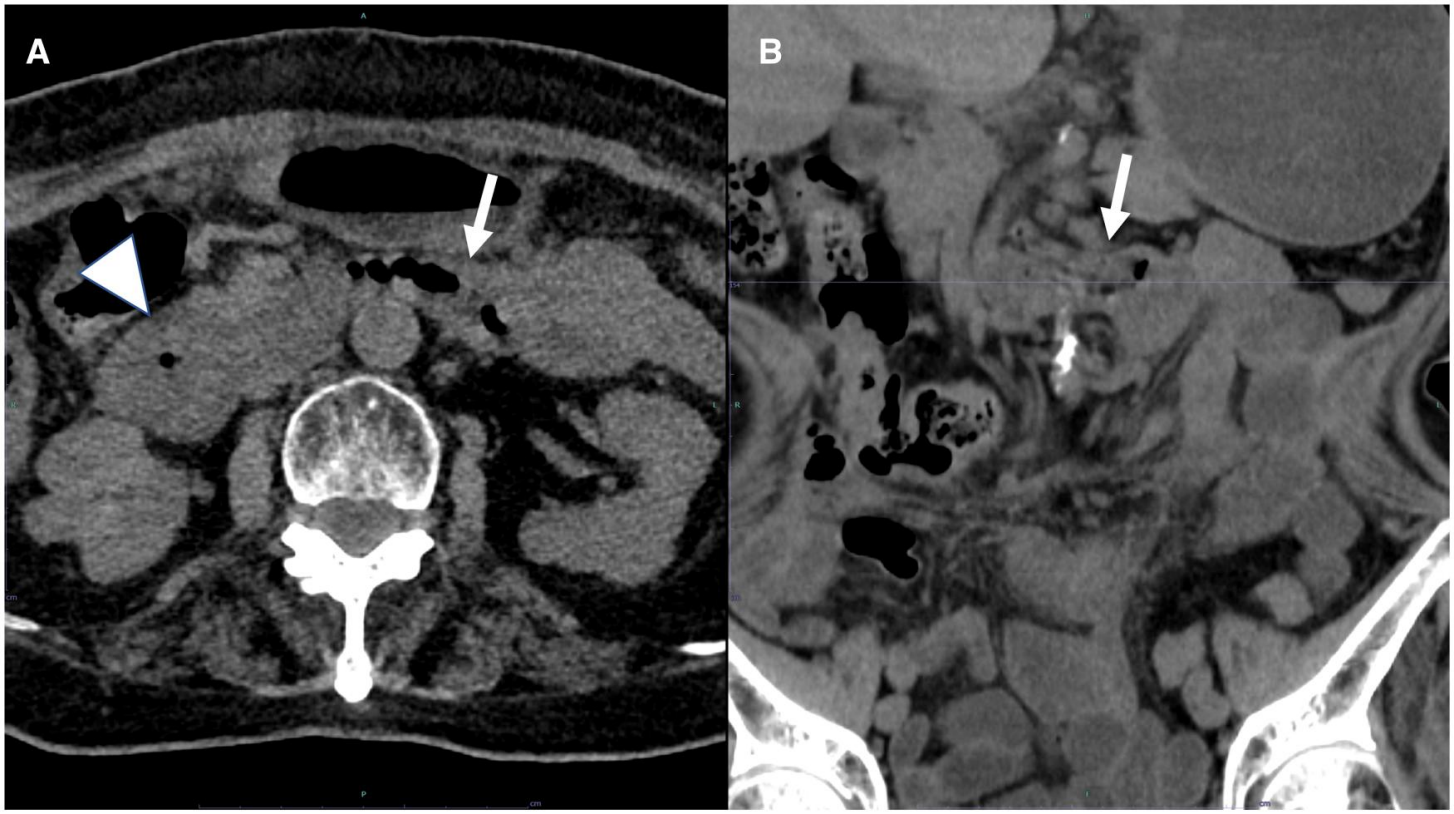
Two months after the second admission, non-contrast-enhanced CT was performed to evaluate nausea and abdominal pain (A, B). The CT images show no abnormalities in the position of the duodenum along its course (arrow). However, oedematous wall thickening is visible in the second part of the duodenum (A, arrowhead).
